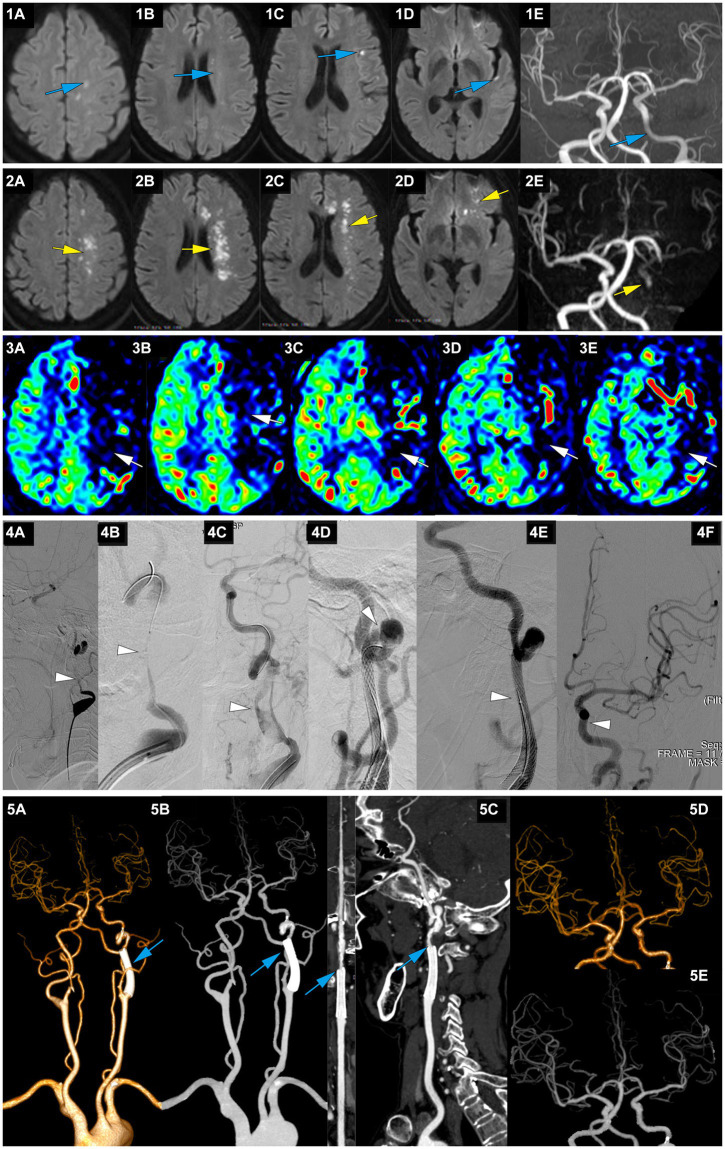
A representative case of AIS with occlusion of LICA caused by dissection. A 63-year-old female with no medical history experienced right limb weakness and slurred speech at 8 am on January 13, 2022. She was able to communicate and walk while holding objects normally (she carried a heavy load on her shoulder 2 days before the onset of symptoms). On January 15, MRI-DWI showed multiple punctate infarctions in the left hemisphere (1A-D, blue), and MRA indicated slightly diminished visualization of the LICA (1E, blue); she was treated with dual antiplatelet therapy of aspirin and clopidogrel. At 7:00 on January 21, her symptoms worsened, presenting as speechlessness and inability to move the right limbs. At 22:30 on January 21, the patient was transferred to our hospital. On admission, the NIHSS score was 12. MRI-DWI indicated a significant increase in the area of infarction (2A-D, yellow), MRA revealed occlusion of the LICA, with faint imaging of the distal MCA (2E, yellow), and ASL showed hypoperfusion in the left cerebral hemisphere (3A-E, white). At 207.3 h after the onset of AIS, emergency angiography showed LICA C1 segment occlusion with mTICI 1 grade (4A, white). A microguidewire and microcatheter were passed through the occlusion (4B, white), and after balloon dilation, a long segment dissection was found in the C1 segment (4C, white). A wallstent 7*40 mm was placed at the proximal end of the C1 segment curvature, but there was still a dissection in the distal end of the stent on angiography (4D, white). Therefore, a Solitaire 6*30 stent was bridged, and angiography showed that the stent completely covered the dissection (4E, white), with mTICI3 grade (4F, white). At discharge, the NIHSS score was 6. At 3 months postoperatively, the NIHSS score was 3, and the mRS score was 2; CTA showed no stenosis in the wallstent stent and mild stenosis at the Solitaire stent site (5A-C, blue), and intracranial vessels were normal (5D-E, blue).
